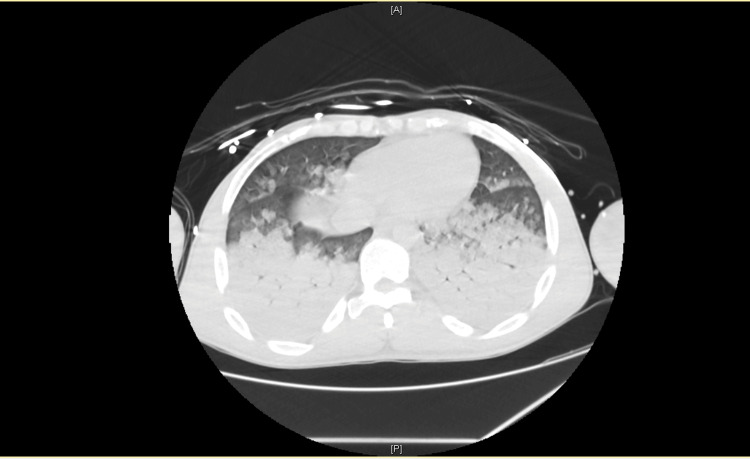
CT revealing large areas of consolidation predominantly affecting the lower lobes.
